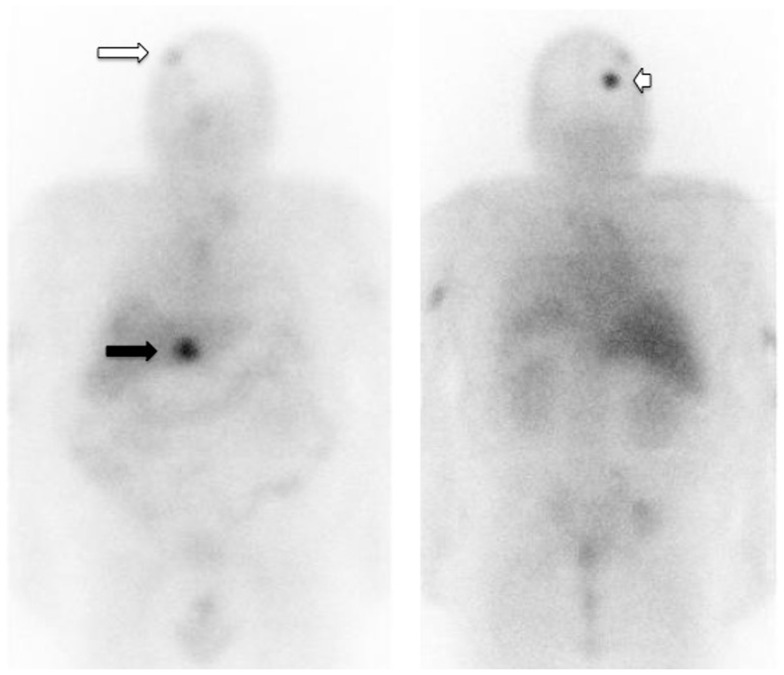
Immunoscintigraphy (anterior and posterior views) recorded in a patient with metastatic medullary thyroid carcinoma. This patient was injected with the anti-CEA hMN14x734 bispecific antibody and a therapeutic activity of 131I-di-DTPA-indium. The immunoscintigraphy recorded 4 days after hapten injection showed a good tumor uptake in liver (black arrow), bone (white arrow), and brain (white arrowhead) metastases.
